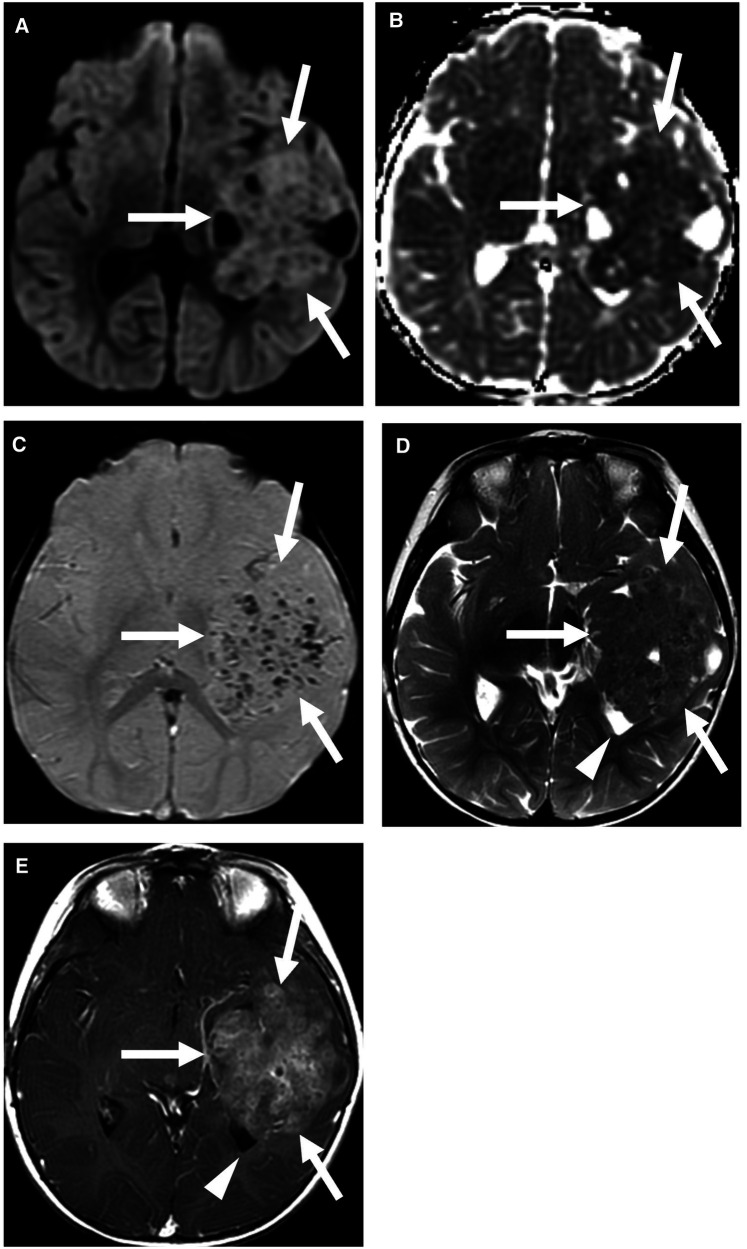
Supratentorial ependymoma, YAP1 fusion-positive. Multiple MRI sequences show a large mass centered in the left temporal lobe (arrows), with partial effacement of the left lateral ventricle (arrowhead). The predominantly solid mass shows restricted diffusion (A,B) and multiple foci of susceptibility (C), which may be related to calcification or prior hemorrhage. The solid component is T2 isointense to the cortex (D) and shows heterogeneous enhancement (E). Case courtesy of Dr. Aashim Bhatia, Children's Hospital of Philadelphia.
